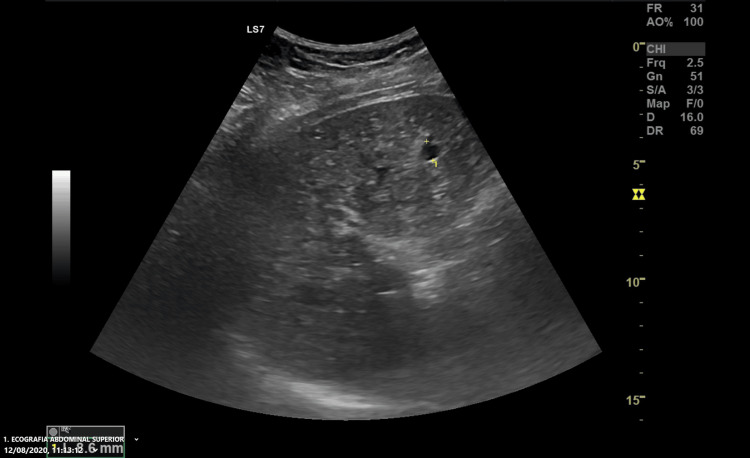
Abdominal Ultrasound Image Abdominal ultrasound revealing a liver with diffusely heterogeneous parenchyma and multiple indeterminate hypoechoic foci (the biggest one in this image measured and labeled with the number 1). This initial imaging study raised the suspicion of Von-Meyenburg complexes.
